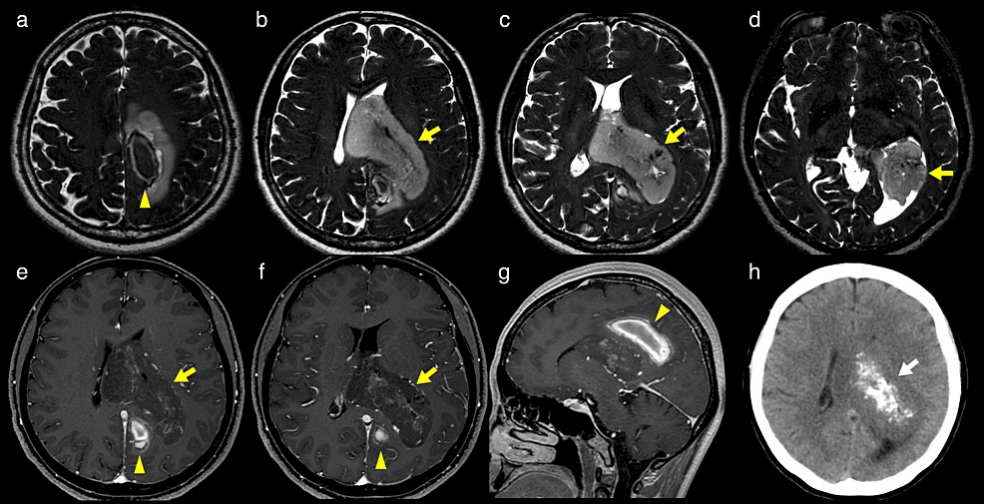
Magnetic resonance imaging and computed tomography Magnetic resonance imaging reveals a T2 hyperintense intraventricular mass with wispy contrast enhancement in the left lateral ventricle (yellow arrow) and T1 hyperintense and T2 hypointense intracerebral hemorrhage at the left cingulate gyrus, which is separated from the intraventricular mass (yellow arrowhead). (a-d) Axial T2 DRIVE images. (e,f) Axial T1-weighted images with gadolinium. (g) Sagittal T1-weighted image with gadolinium. (h) Axial computed tomography image shows intra-tumoral calcification (white arrow).
